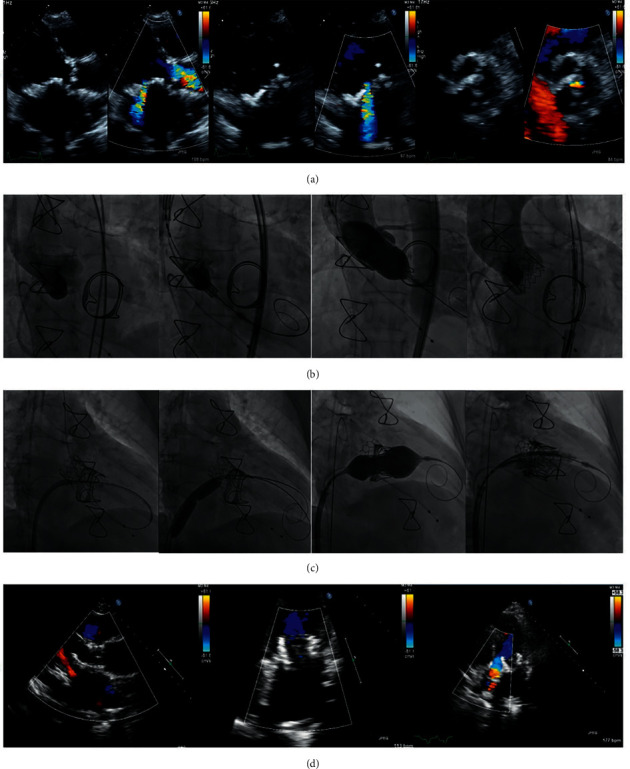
Transcatheter mitral valve in a degenerated Carpentier–Edwards (Edwards Lifesciences) valve (TMViV) with a concomitant transcatheter aortic valve implantation (TAVI). A 64-year-old, diabetic, hypertensive female patient, with COPD and renal transplant, had a degenerated bioprosthetic 29 mm Carpentier–Edwards MV and a degenerated calcific AV with an associated AF. (a) TTE shows a degenerated bioprosthetic MV (severe MS and severe MR) with a calcific degenerated AV (severe AS and mild AR). (b) Fluoroscopy shows a transaortic TAVI of an Edwards SAPIEN-3 23 mm valve during rapid pacing. (c) Fluoroscopy shows an 8.5F-agilis™ sheath including a 5F-MP catheter over a 0.035-inch curved Terumo guidewire to cross the degenerated bioprosthetic MV to the LV, and then to the aorta. Balloon dilatation of the transseptal puncture using a 14 mm balloon over a 0.035-inch/260 extra-stiff Confida™ guidewire. Transseptal TMViV of an Edwards SAPIEN-3 29 mm valve. (d) TTE: both SAPIEN-3 valves are in mitral and aortic positions with normal flow across both valves. AF: atrial fibrillation, AR: aortic regurgitation, AS: aortic stenosis, AV: aortic valve, AVA: aortic valve area, COPD: chronic obstructive pulmonary disease, LV: left ventricle, MR: mitral regurgitation, MS: mitral stenosis, MV: mitral valve, MVA: mitral valve area, Pg: pressure gradient, TAVI: transcatheter aortic valve implantation, TMViV: transcatheter mitral valve-in-valve, and TTE: transthoracic echocardiography.
